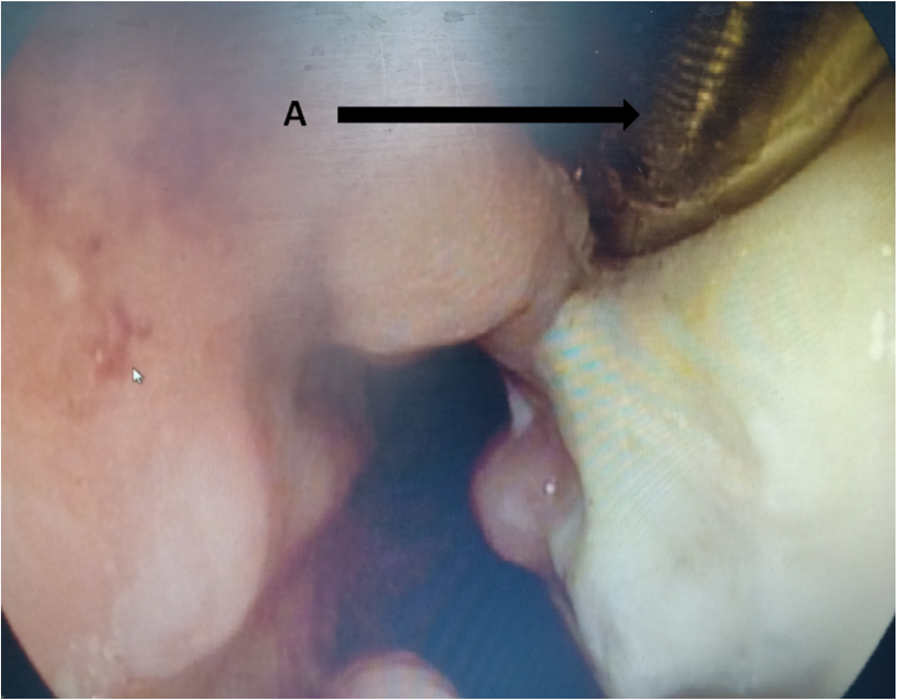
The gastroscopy after removal of sump drainage tube. a Nasogastric sump drainage tube (a) was inserted through the fistula with the guidance of radiography or gastroscopy and the distal tip of the tube was positioned at the bottom of the abscess cavity. b Naso-jejunum three lumen feeding tube (b) was placed under the radiographic or gastroscopic guidance. The functions of this tube include nasogastric decompression and nasojejunum enteral nutrition. c The closed fistula
